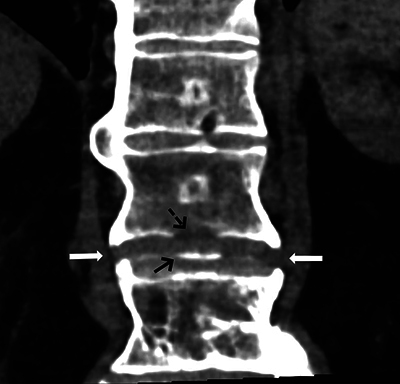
Coronal CT-scan image showing the fractured syndesmophytes (arrows) alongside a partial avulsion of the inferior endplate (black arrow) of T12 corresponding to the cortical defect (dotted arrow).
